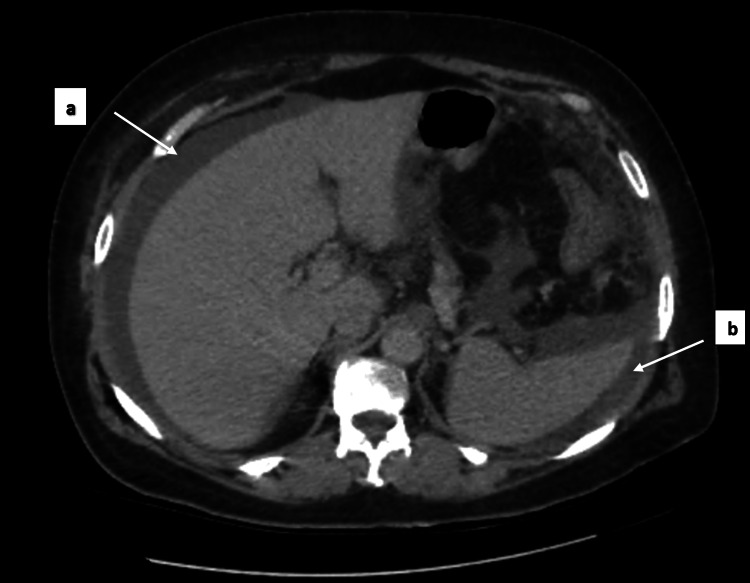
Axial CT scan showing perihepatic (a) and perisplenic (b) fluid collections
